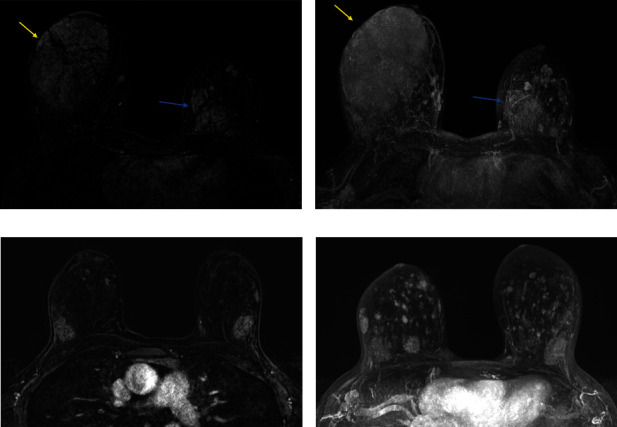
A 39-year-old woman with symptomatic PASH. Contrast-enhanced axial MRI (a) and maximum intensity projection (MIP) (b). Images show a right outer central breast 9 : 00 anterior to a middle depth 10.1 cm heterogeneously enhancing oval mass with circumscribed margins and interspersed areas of fat (arrow), biopsy-proven PASH. The left breast also has a lower inner quadrant 7 : 00 posterior depth 4.1 cm heterogeneously enhancing oval mass with circumscribed margins (thin arrow), also later found to be biopsy-proven PASH. Approximately two years later, after excision of these two masses and treatment with a course of tamoxifen, the patient developed diffuse PASH in both breasts as seen on contrast-enhanced axial MRI (c) and MIP (d) images.
